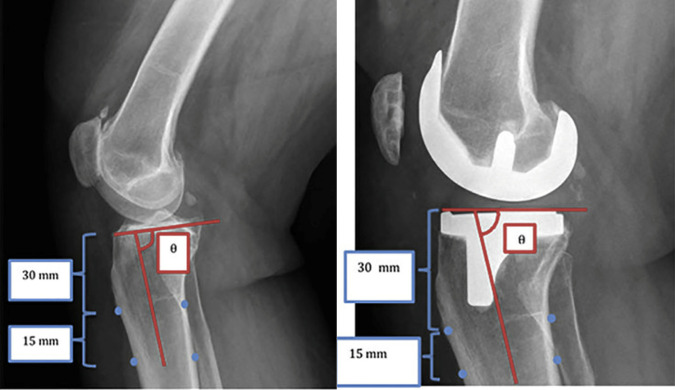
Radiographic determination of PTS in a native knee and TKA. Tibial slope (θ) was determined by the angle formed from a line bisecting the tibial metaphysis and a line tangential to the tibial joint surface on a lateral radiograph (red lines). PTS = posterior tibial slope, TKA = total knee arthroplasty
